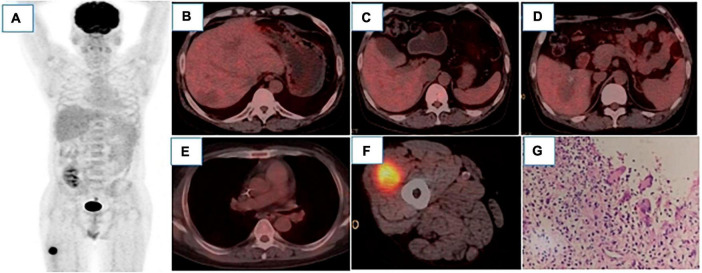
18 F-FDG PET/CT MIP image (A) showed only hypermetabolic lesions on the right thigh. After 6 cycles of chemotherapy, there were still low-density lesions in the liver, but there was no increase in FDG uptake, which were as follows: Segment VI, with the size of 27 × 22°mm and SUVmax 2.2 (B), segment I, with the size of 38 × 29°mm and SUVmax of 1.8 (C), segment VIII, with the size of 42 × 30°mm and SUVmax of 2.2 (D), liver SUVmax was 2.3. The left main bronchus wall was slightly thickened without significant FDG uptake (E). In the vastus lateralis muscle of the right thigh, nodular slightly low-density shadow was observed with the size of 32 × 28°mm, FDG uptake was increased with the SUVmax of 8.8. (F) Pathology [(G), HE400 × ] showed fibrous hyperplasia around necrotic tissue, accompanied by inflammatory cells and multinucleated giant cells reaction, which was considered as necrotizing granulomatous inflammation.
